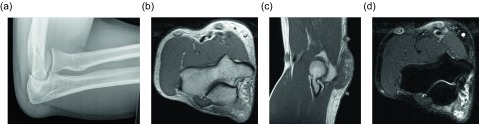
Lipoma arborescens: 42-year-old male with prominent soft tissue over olecranon since injury one year ago. Lateral radiograph (a) of the elbow shows fullness of the olecranon bursa without underlying osseous abnormality. Axial (b) and sagittal (c) T1W and T2W FS (d) images demonstrate frond-like, fatty hypertrophy of the synovium of the olecranon bursa.
